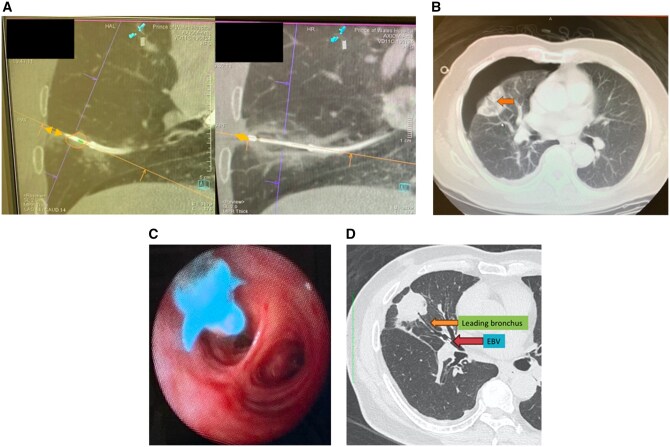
(A) Intraoperative CBCT showing significant tissue contraction and a shift in the position of the catheter. The catheter shifted closer to the visceral pleura after TMWA. (B) CT was performed as the patient developed a pneumothorax immediately after the procedure. The CT showed a single BPF following the ablation tract from a single leading airway (orange arrow). (C) Bronchoscopic EBV placement into the leading airway was performed. Air leak ceased immediately after EBV placement. (D) An interval CT was performed at 1 month, showing a contracted ablation zone and a healing BPF coming from the leading airway (orange arrow). EBV was in situ (red arrow). Abbreviations: BPF, bronchopleural fistula; CBCT, cone-beam CT; EBV, endobronchial valve; TMWA, transbronchial microwave ablation.
